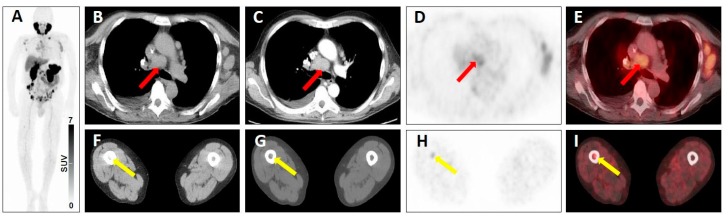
Equivocal uptake in soft-tissue and bone lesions on PSMA-targeted positron emission tomography (PET)/computed tomography (CT). ^68^Ga-PSMA-11 PET/CT for restaging in a 66-year-old man with a history of a prostate cancer. Whole-body maximum intensity projection image showing multiple metastases to lymph nodes and to the bone with both clearly pathologic radiotracer uptake and equivocal radiotracer uptake (A). Axial low-dose CT image (B), contrast-enhanced CT image (C), PET image (D), and fused PET/CT image (E) showing a mediastinal lymph node typical of a prostate cancer lesion on anatomic imaging, but with equivocal uptake (red arrows), consistent with a PSMA-RADS-3A lesion. Axial soft-tissue window CT image (F), bone-window CT image (G), PET image (H) and fused PET/CT image (I) showing a bone marrow lesion not atypical of a prostate cancer lesion on anatomic imaging, but with equivocal uptake (yellow arrows), consistent with a PSMA-RADS-3B lesion.
